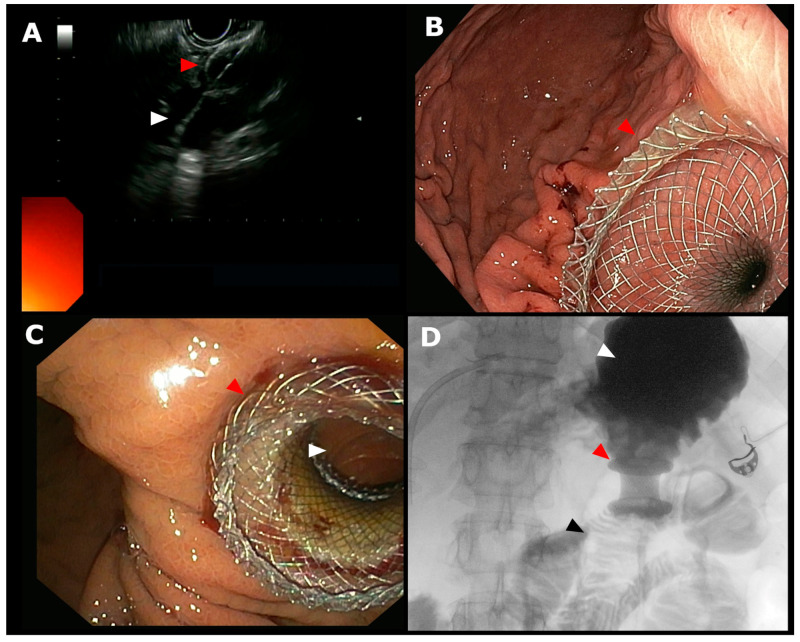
(A) Endoscopic ultrasound (EUS) view of lumen-apposing metal stent (LAMS) system deployment with distal tip into the jejunal lumen (white arrow) and distal flange released into the jejunum (red arrow). (B) Endoscopic view of proximal flange of LAMS into the gastric cavity when LAMS is not dilated. (C) Endoscopic view of a gastrojejunostomy (GJ) after dilation of the LAMS; white arrow indicates jejunal lumen and red arrow indicates proximal flange of the LAMS. (D) Radiologic evaluation of the GJ showing contrast dye in the stomach (white arrow) flowing through the LAMS (red arrow) into the jejunum (black arrow).
